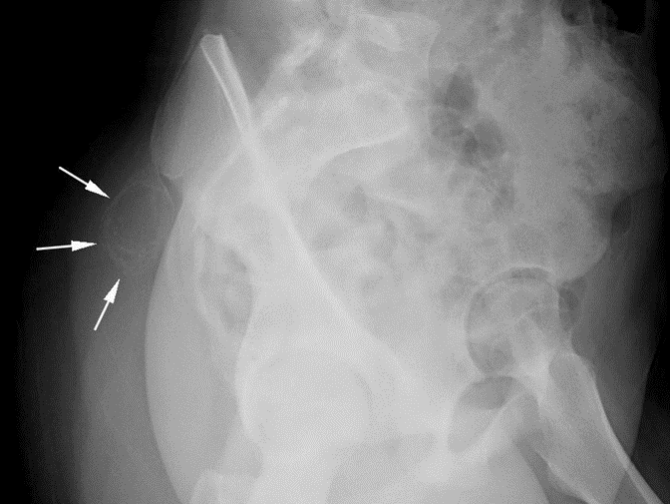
X-ray pelvis – typical peripheral ossification of the mature lesion (arrows).
